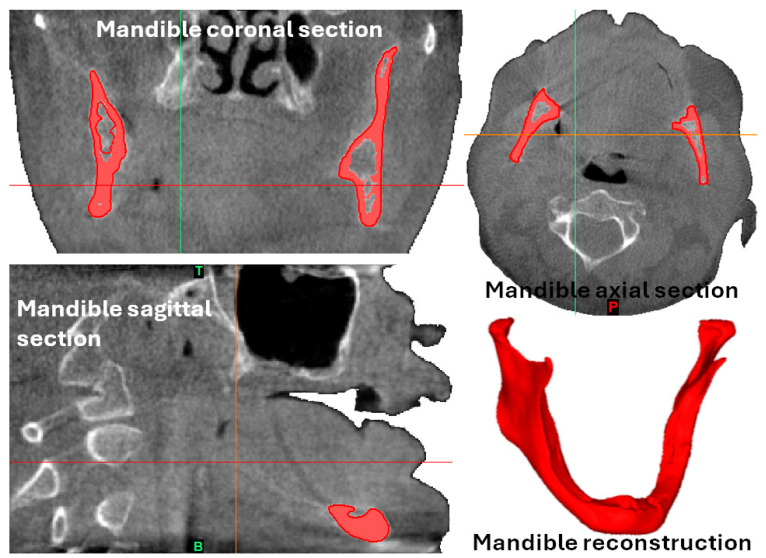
Patient CT-scan images with maxilla and mandible reconstructions.
